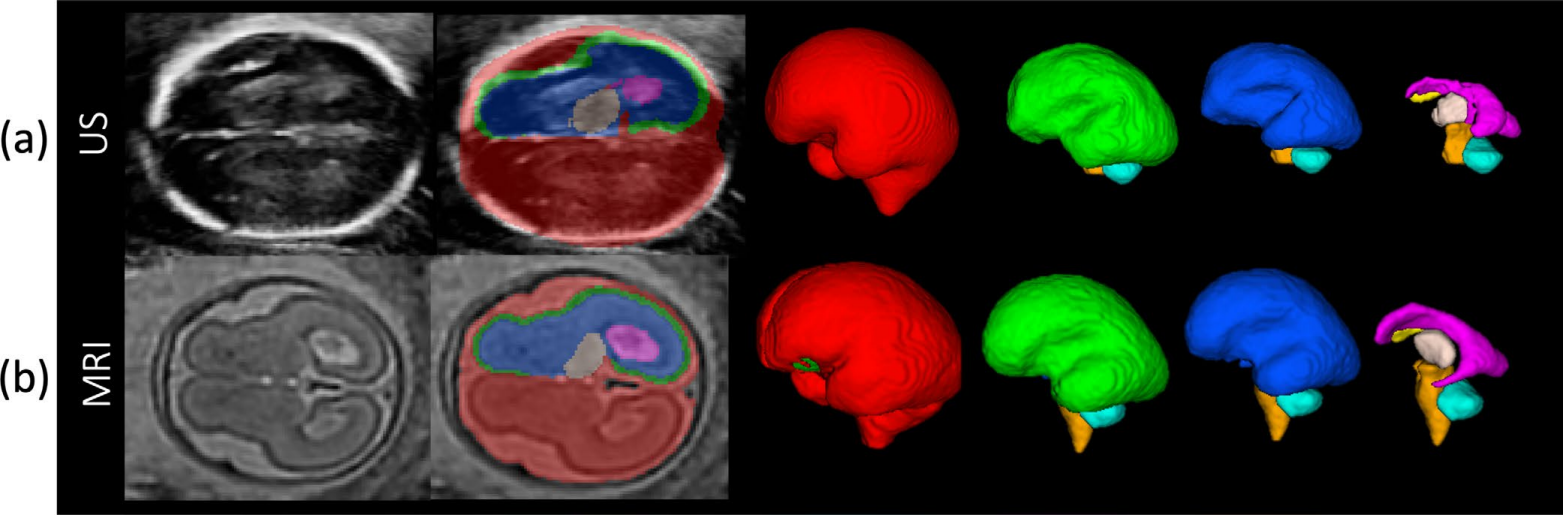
An overview of the segmentations from (a) US and (b) MRI. The ICV is shown in red, CoP in green, WDGM in blue, ventricular system in pink, Th in beige, CSP in yellow, CB in cyan, and BS in orange. Tissues are only segmented in the distal hemisphere (the hemisphere furthest from the probe), except ICV which is extracted across both hemispheres.
